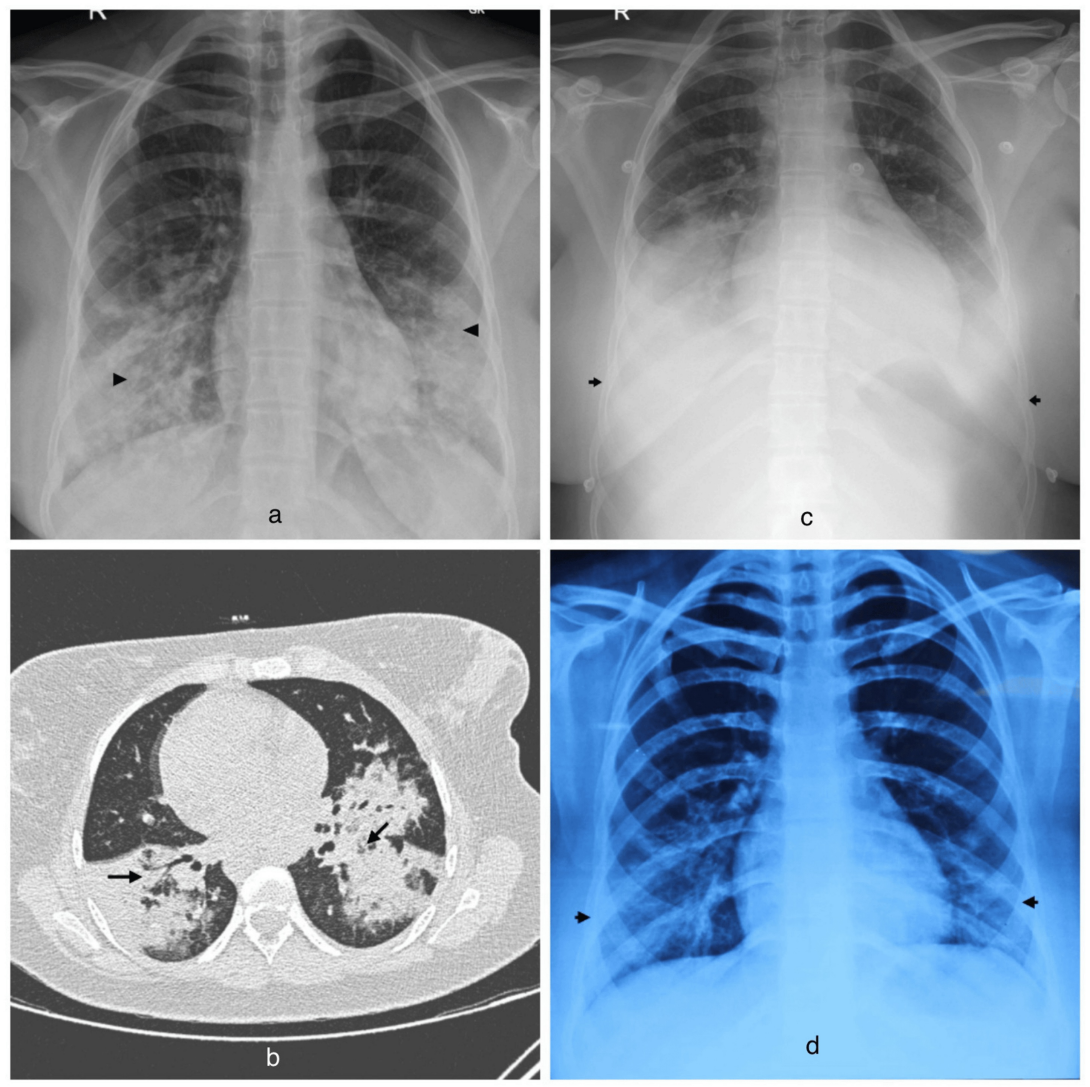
a. Chest X-ray on the day of admission (7th day of illness), b. High-resolution computed tomography of the chest, c. Chest X-ray on the 14th day of illness, d. Chest X-ray obtained two weeks after discharge a. Chest X-ray on the day of admission, which is the 7th day of illness, shows confluent areas of consolidation in the bilateral lower lung zones (black arrowheads). b. High-resolution computed tomography of the chest reveals bilateral large confluent areas of dense consolidation with air bronchograms, mainly involving the lower lobes (black arrows), along with a few patchy dependent areas in the right upper lobe. There is an associated bilateral mild pleural effusion, more pronounced on the right side, and mildly enlarged, discrete, non-calcified mediastinal lymph nodes. The findings suggest a likely infective etiology. c. Chest X-ray on the 14th day of illness demonstrates bilateral lower zone confluent opacities and consolidation, with an obscured costophrenic angle (black arrows). d. Chest X-ray obtained two weeks after discharge shows resolution of bilateral lower zone opacities (black arrows) and a clear, bilaterally normal costophrenic angle.
